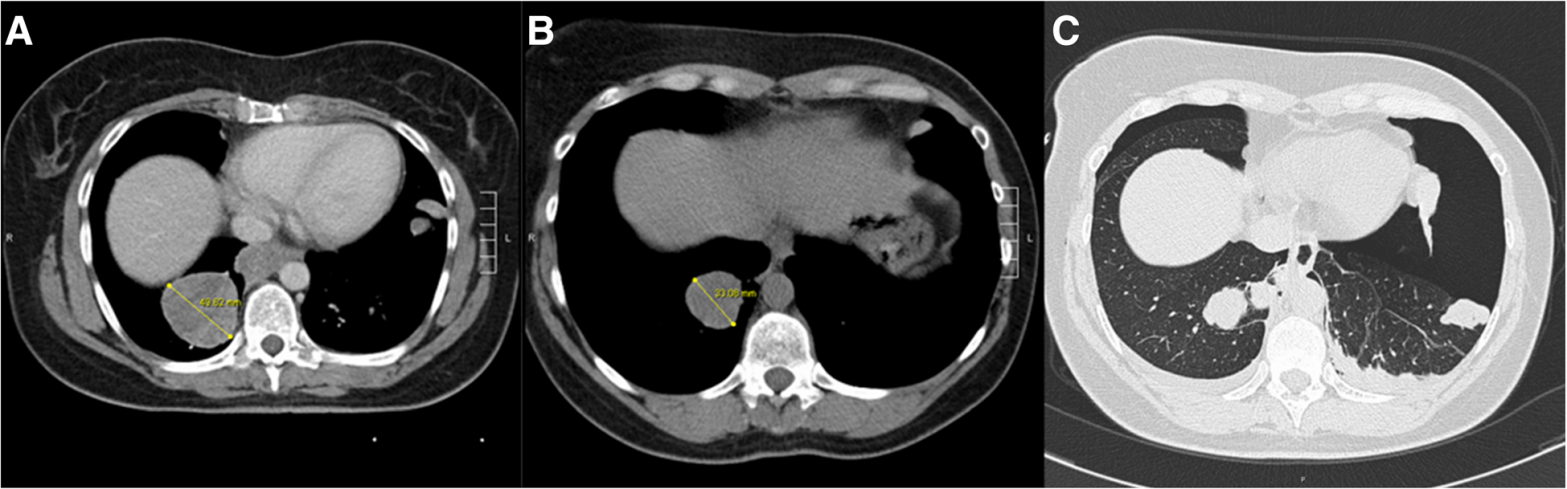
CT scan of patient 6. A: CT scan at start of pazopanib showing a metastasis in the right lower lobe with a diameter of 49.6 mm. B: CT scan after 12 weeks of pazopanib (6 weeks of treatment and 6 weeks on hold due to liver toxicity), showing a decrease in diameter of the metastasis to 33.1 mm. C: CT scan showing the bilateral pneumothorax.
